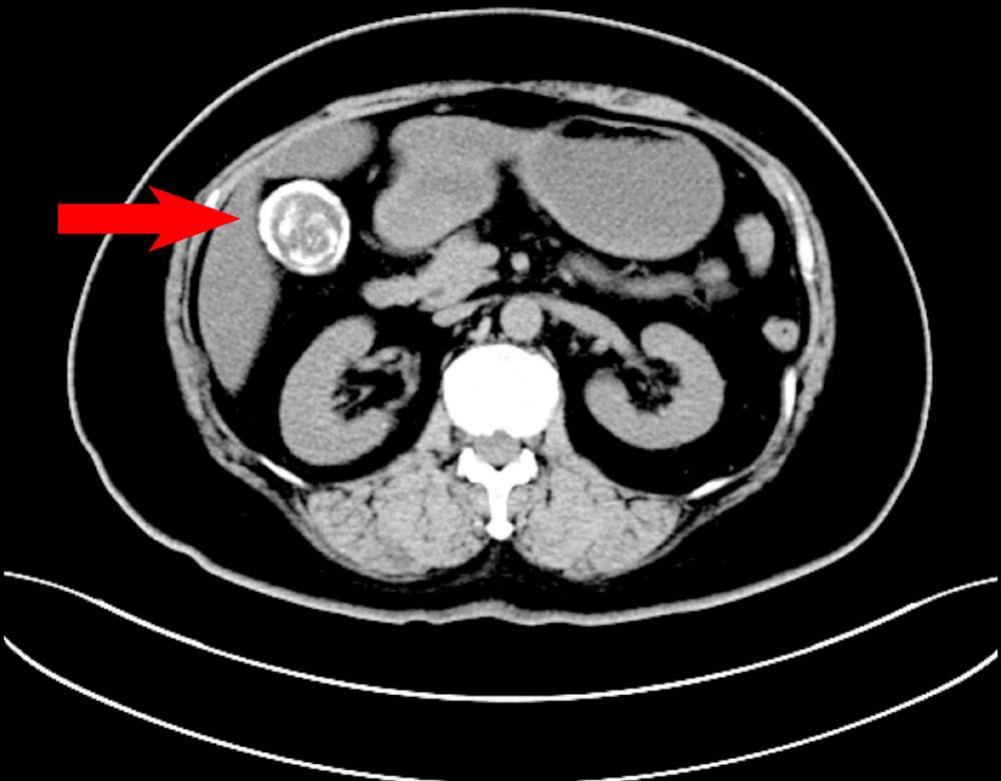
The latest general CT of the abdomen revealed an eggshell-shaped, evenly thickened cholecystic wall witha ring, high-dense shadow, suggesting diffuse calcification of the whole gallbladder wall classically associated with porcelain gallbladder. CT = computed tomography.
